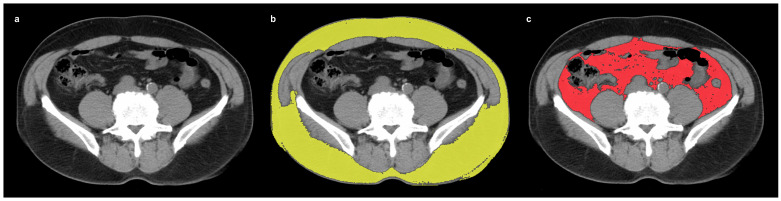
An example of measuring the CT-attenuation of SAT and VAT. On a transaxial CT image at the level of the L4–L5 vertebrae (a), SAT and VAT areas were automatically selected using a CT-attenuation threshold. SAT (b) was defined as extra-peritoneal adipose tissue with a CT-attenuation threshold between −190 and −30 HU (yellow area). VAT (c) was defined as the intra-abdominal adipose tissue between a CT-attenuation threshold of −150 and −50 HU (red area). (CT, computed tomography; HU, Hounsfield units; SAT, subcutaneous adipose tissue; VAT, visceral adipose tissue).
